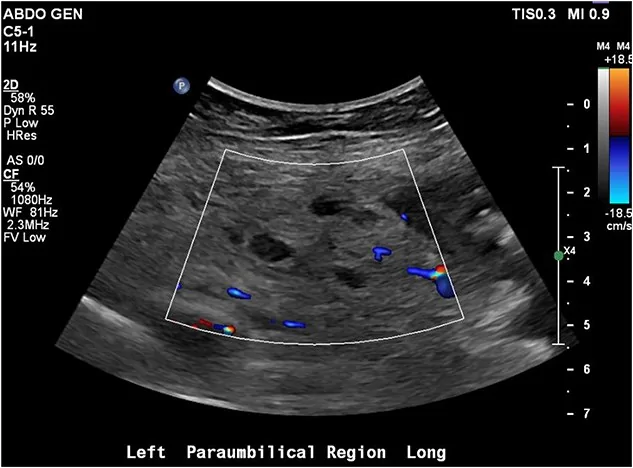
Longitudinal ultrasound image of the left paraumbilical region on Day 3 demonstrating a 40 × 28 × 36 mm non-peristaltic fluid- and gas-containing structure surrounded by echogenic inflamed mesentery and adjacent reactive mesenteric lymphadenopathy. Correlation with CT findings raised suspicion for jejunal diverticulitis with possible localized abscess formation.
